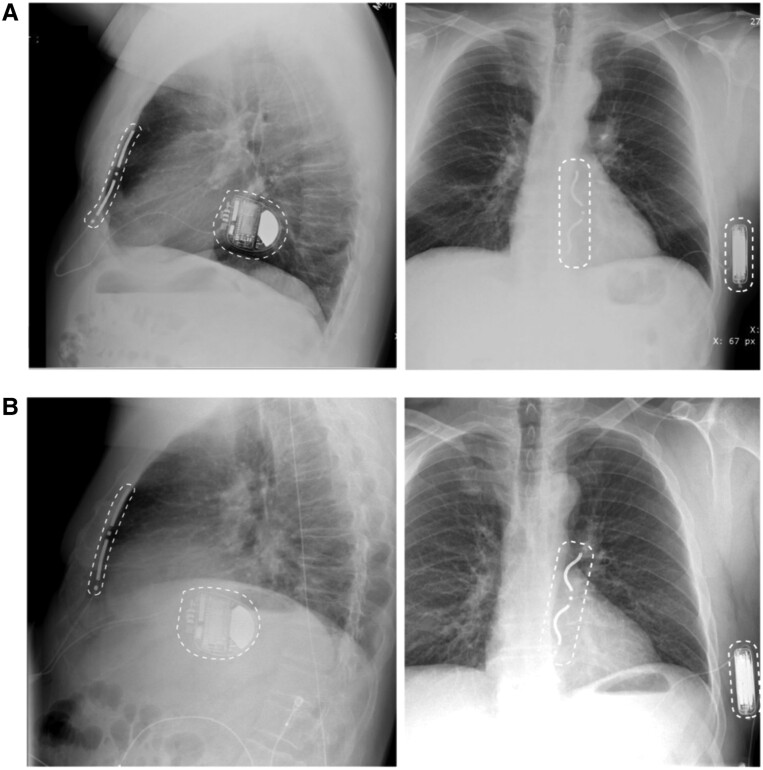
X-ray imaging of original EV ICD implant before extraction and replacement EV ICD system. Lateral and frontal X-rays of the original EV ICD implant before extraction (*A*). Lateral and frontal X-rays of replacement EV ICD that was implanted after extraction 35.9 months post-implant (*B*). The can and epsilon lead tip are indicated with a dashed outline.
